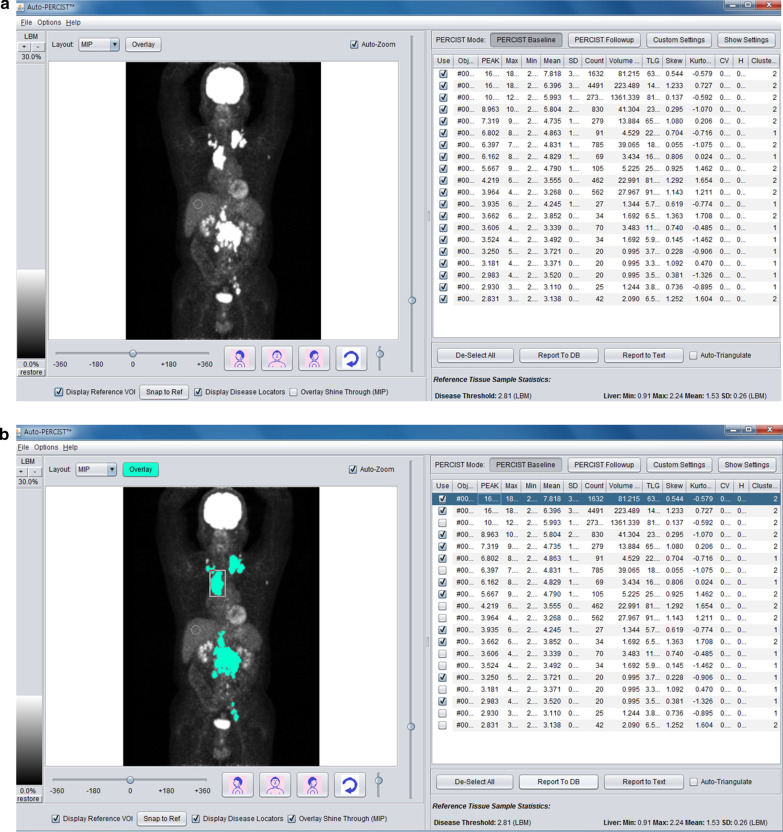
Screen captures from Auto-PERCIST™. a The software detected all sites with SUL_peak_ higher than the computed or manually set threshold. b The reader than selected the true tumor lesions (shaded in green), excluding physiologic [^18^F]FDG activity
